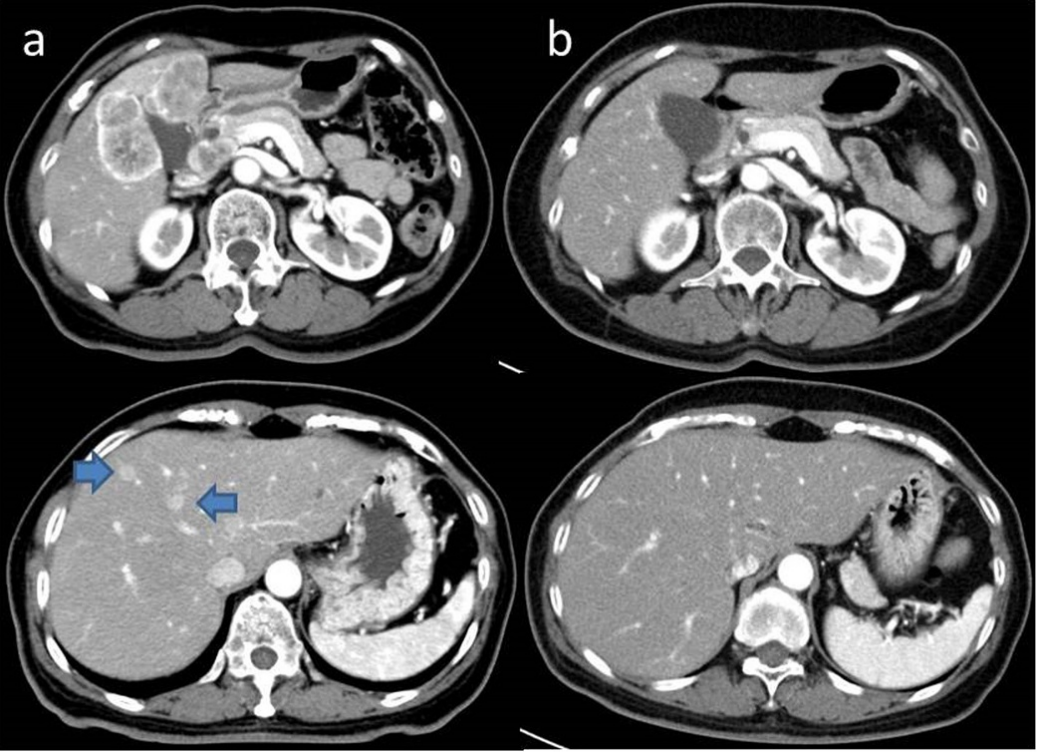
Changes in CT images of the gallbladder cancer and liver metastases: (a) before chemotherapy; (b) after four cycles of chemotherapy.
